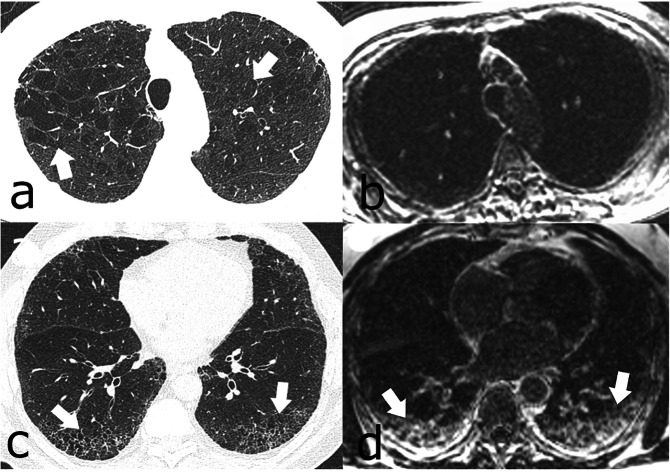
Fifty-seven-year-old man with progressive breathlessness, FEV_1_ 2.4 L/s, LAV 7.6%. a Axial high-resolution computed tomography showed moderate centrilobular emphysema in the upper lobes (arrows). b It was unclear at multidisciplinary conference whether the abnormal regions in the lower lobes (arrows) represented emphysema or fibrosis. c Late gadolinium-enhanced magnetic resonance imaging of the upper lobes showed no contrast enhancement, but in d, definite contrast enhancement in the abnormal regions in the lower lobes (arrows) was consistent with fibrosis. *FEV*_*1*_, Forced expiratory volume in one second; *LAV%*, Low attenuation value percent
